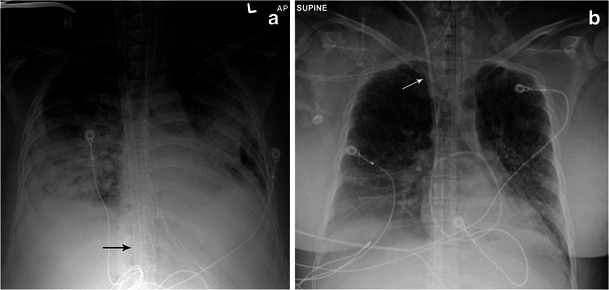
Malpositioned cannulas. Chest radiograph of a 54-year-old male status post MI on peripheral VA ECMO a demonstrates the tip of a superior approach venous ECMO cannula projecting too inferiorly over the IVC (*thin arrow*). A chest radiograph from a different patient b shows the tip of an inferior approach venous cannula projecting over the lower SVC, much higher than the expected location of the right atrium
